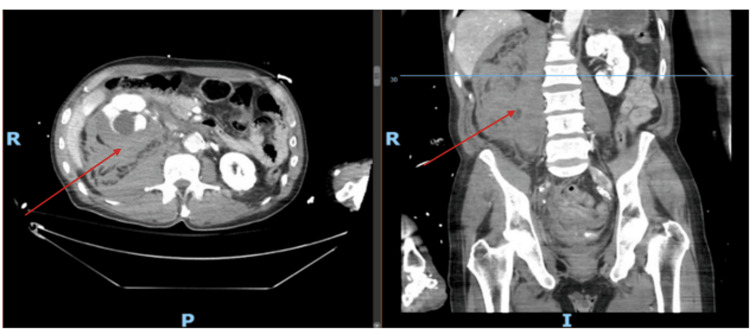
Repeat CT scan (axial image on the left and sagittal image on the right) after the patient had abdominal pain after heart catheterization. Renal subcapsular hematoma measuring 10×9×7 cm is shown. Retroperitoneal hemorrhage is present too (see red arrows). Axial and sagittal views are shown.
